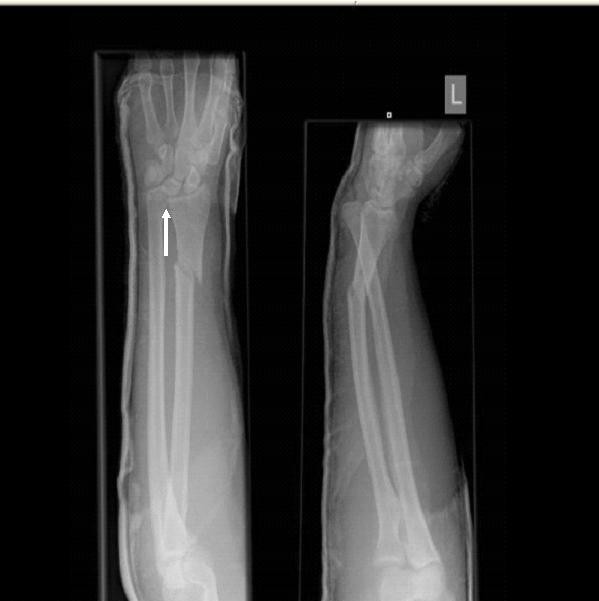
Radiographs at initial presentation show a comminuted fracture of the right radial shaft (junction of middle and distal thirds) and distal radioulnar joint (DRUJ) dislocation but no evidence of elbow dislocation.
